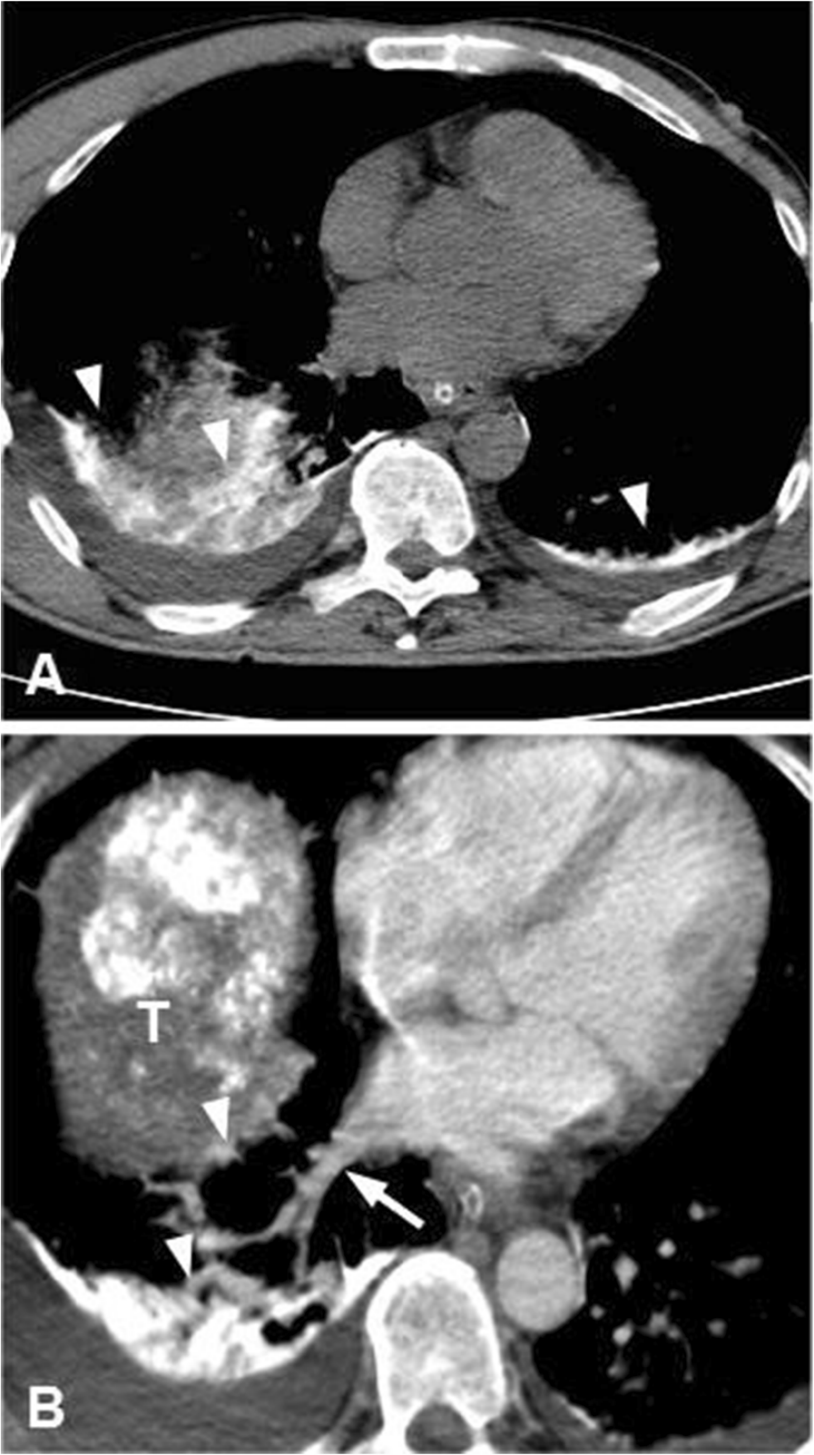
Extensive pulmonary embolization and dilated pulmonary vessels could be observed post embolization. (A) A follow-up CT performed 3 days post TAE showed extensive lipiodol embolization (arrowheads) in both lungs well visible in the atelectatic basal segments. (B) In the vicinity of the right diaphragmatic surface, widened vascular spaces (arrowheads) and a thick pulmonary vein (arrow) could be identified, suggestive of a pulmonary AV shunt.
